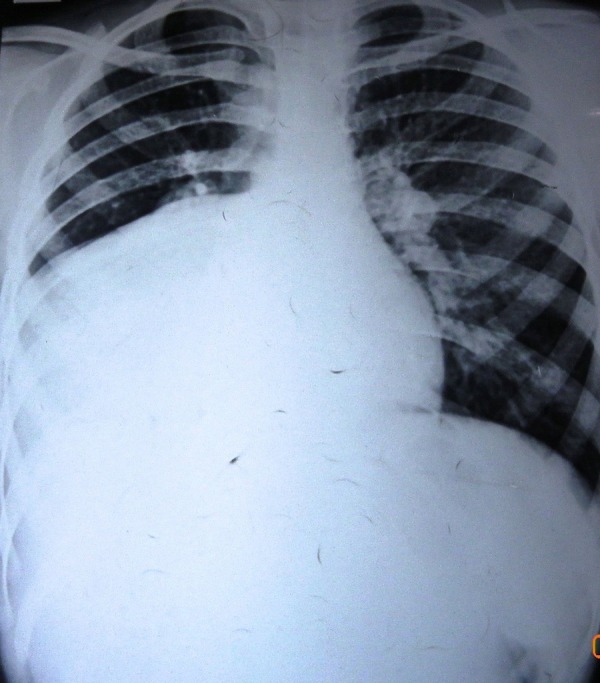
Figure 1: Plain Radiograph of the chest showing a 10 x 10cm opacity in the right lower zone of lung.
